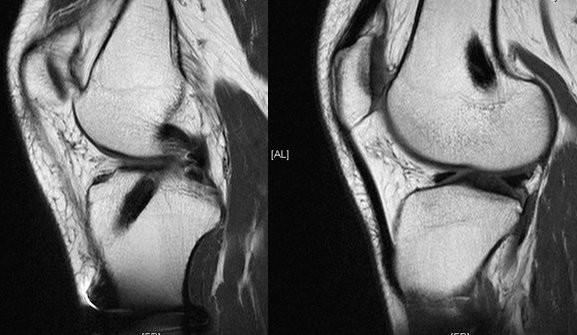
MR image of the tendon graft in the bone tunnel showed excellent incorporation.
